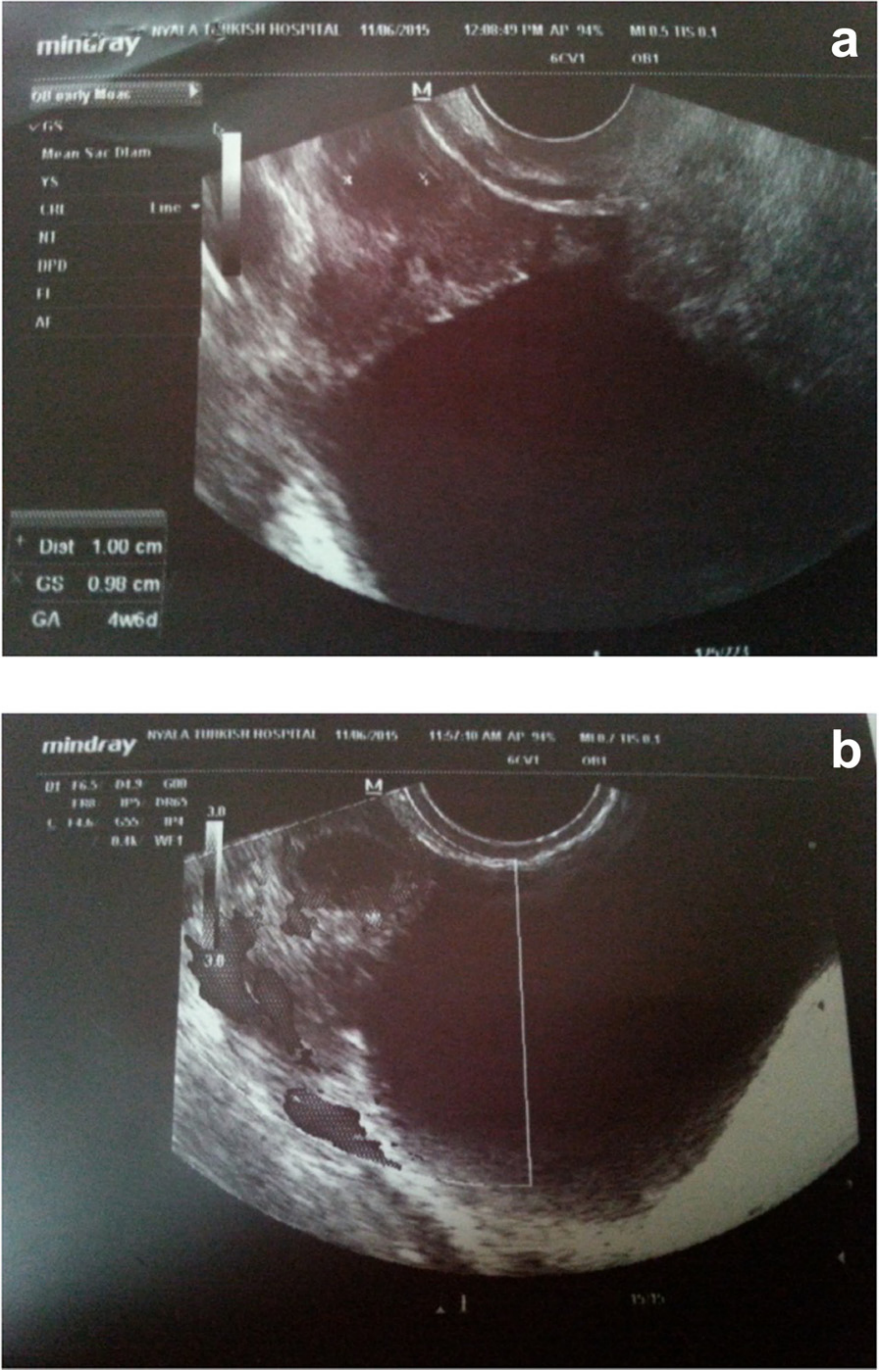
Ultrasound images of ovarian gestational sac before medical methotrexate therapy. (a) Ovarian gestational sac (b) Colour doppler image of gestational sac
